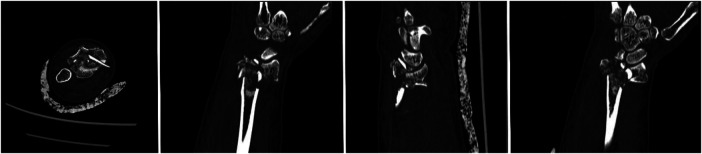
Patient's preoperative CT scan of the fracture.
